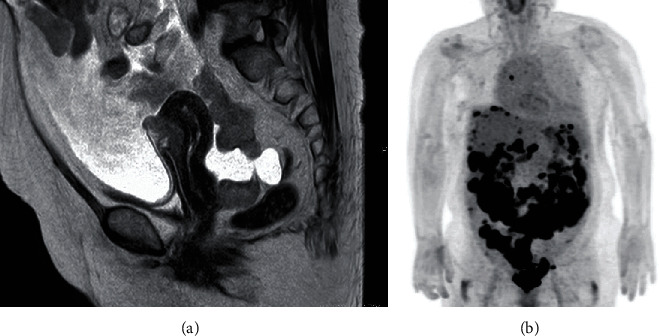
(a, b) T2-weighted magnetic resonance imaging of the pelvis and 18F-fluoro-2-deoxy-d-glucose positron emission tomography/computed tomography revealed massive peritoneal dissemination, including in the Douglas' pouch, vesicouterine pouch, omentum, and the surface of the liver and spleen. Bilateral ovaries were not clearly enlarged and were not visualized in the image.
